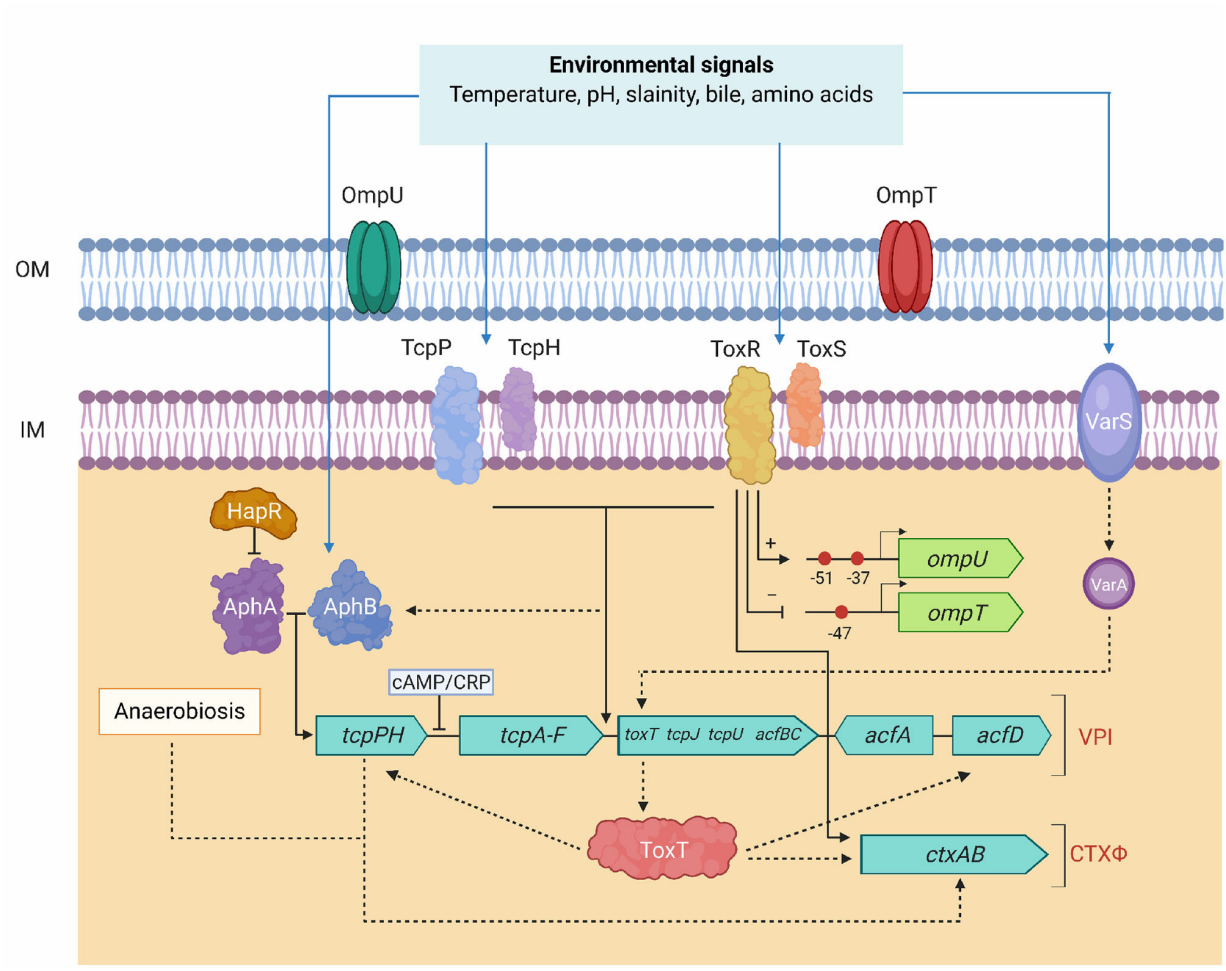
Regulation model of Tox-mediated virulence genes in *V. chlerae*. TcpP/TcpH and ToxR/ToxS function together in activating transcription of *toxT*. ToxR and TcpP need accessory proteins (ToxS and TcpH, respectively) for maximal activity. ToxT initiates transcription of the full *tcp* operon, *ctxAB*, and *acf*. AphA and AphB activate transcription of the *tcpPH* operon in response to environmental conditions. *tcpPH* promoter is negatively influenced by the global regulator, CRP. ToxR/ToxS, and AphA/AphB also regulate genes other than *ctx* and *acf*. ToxRS, AphAB, TcpPH, and ToxT coordinately regulate the transcription of *ctx* and *tcp*. AphA and AphB activates expression of the *tcpPH*. TcpPH and ToxRS regulate *ctx* and *tcp* genes through ToxT. AphB has been linked to the expression of *tcpPH* for anaerobiosis that enhances the production of CT. The direct activator and the second activator are differentiated by black solid and dotted arrows, respectively. Blue arrows indicate different environmental conditions sensed by the ToxRS and TcpPH, AphAB, and VarS regulatory systems. The two-component VarS-VarA system responds to environmental factors and signals *toxT*. ToxR, independently activates and represses transcription of *ompU* and *ompT*. ToxR modulation was shown by an arrow (*ompU*) and line with base (*ompT*) through + for the transcription of *ompU* and—for repressing the transcription of *ompT*. Red circles in *ompU* and *ompT* indicate the ToxR binding sites and the transcriptional start sites are marked with arrows for each promoter.
